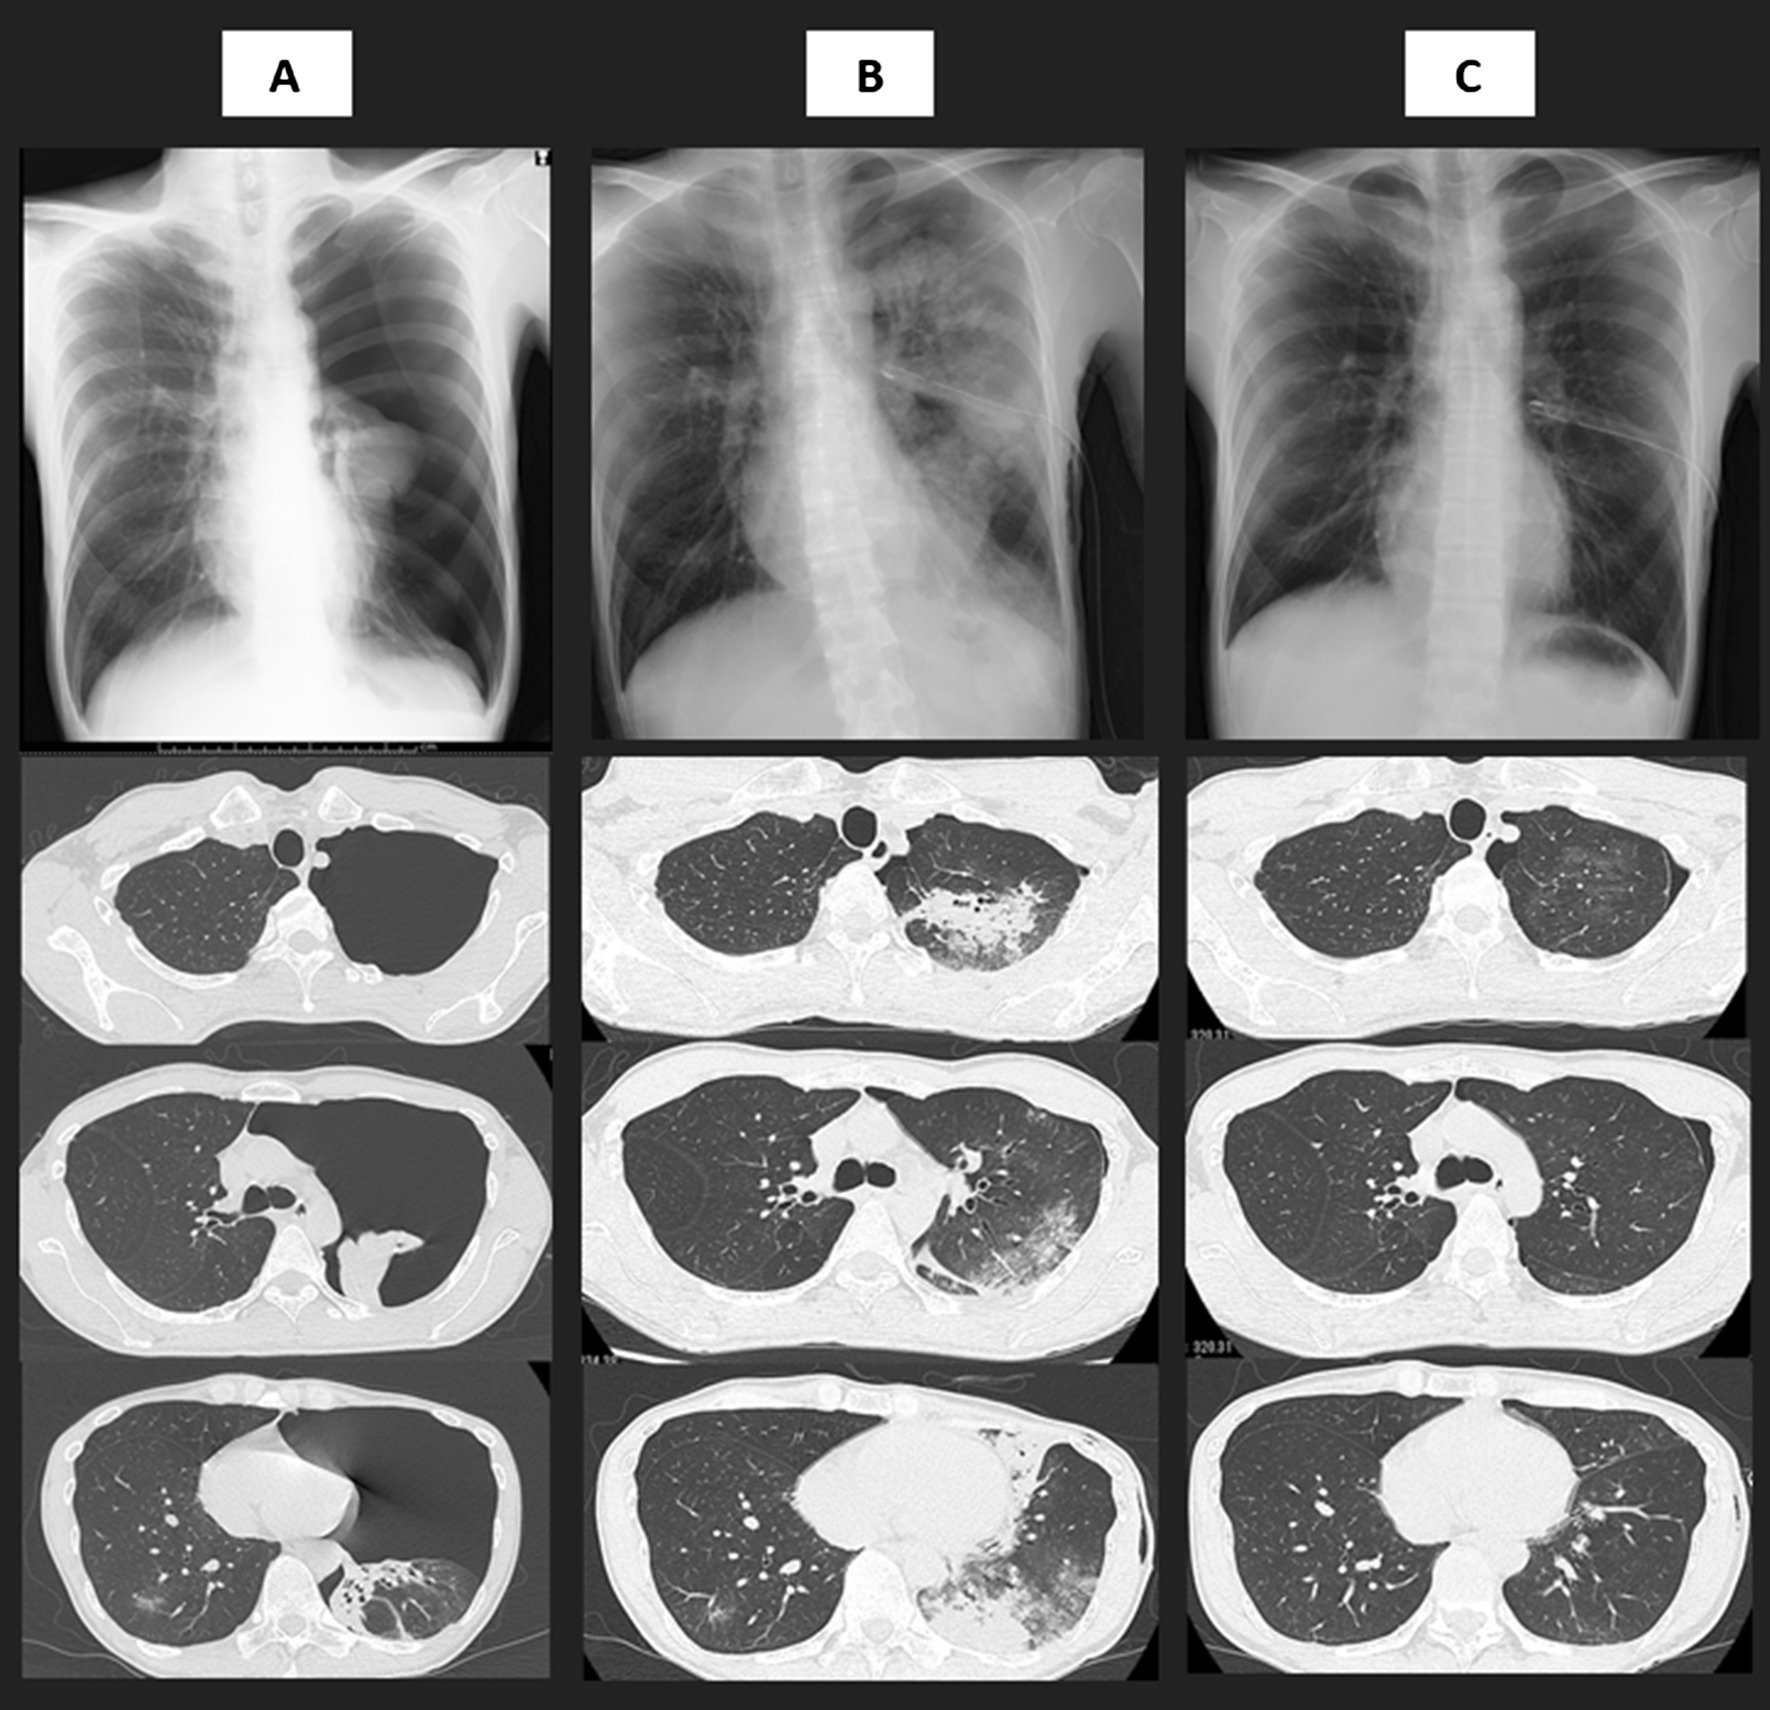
Chest X-ray and thin-section computed tomography at upper lobes, carina and lower lobes levels on admission (A), 1 day after thoracic drainage procedure (B) and 7 days after thoracic drainage procedure (C)
